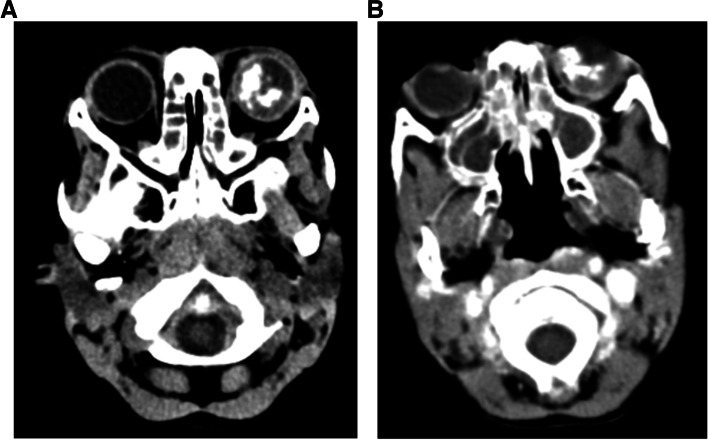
A An unenhanced axial CT image at the level of the orbits. There was a left orbital mass with calcifications involving the vitreous. The mass measured 2.1 × 1.8 cm. B A contrast enhanced axial CT, which demonstrated the same features as on A. There was no enhancement of the left vitreous mass. A diagnosis of a left orbital retinoblastoma was made on A and B
